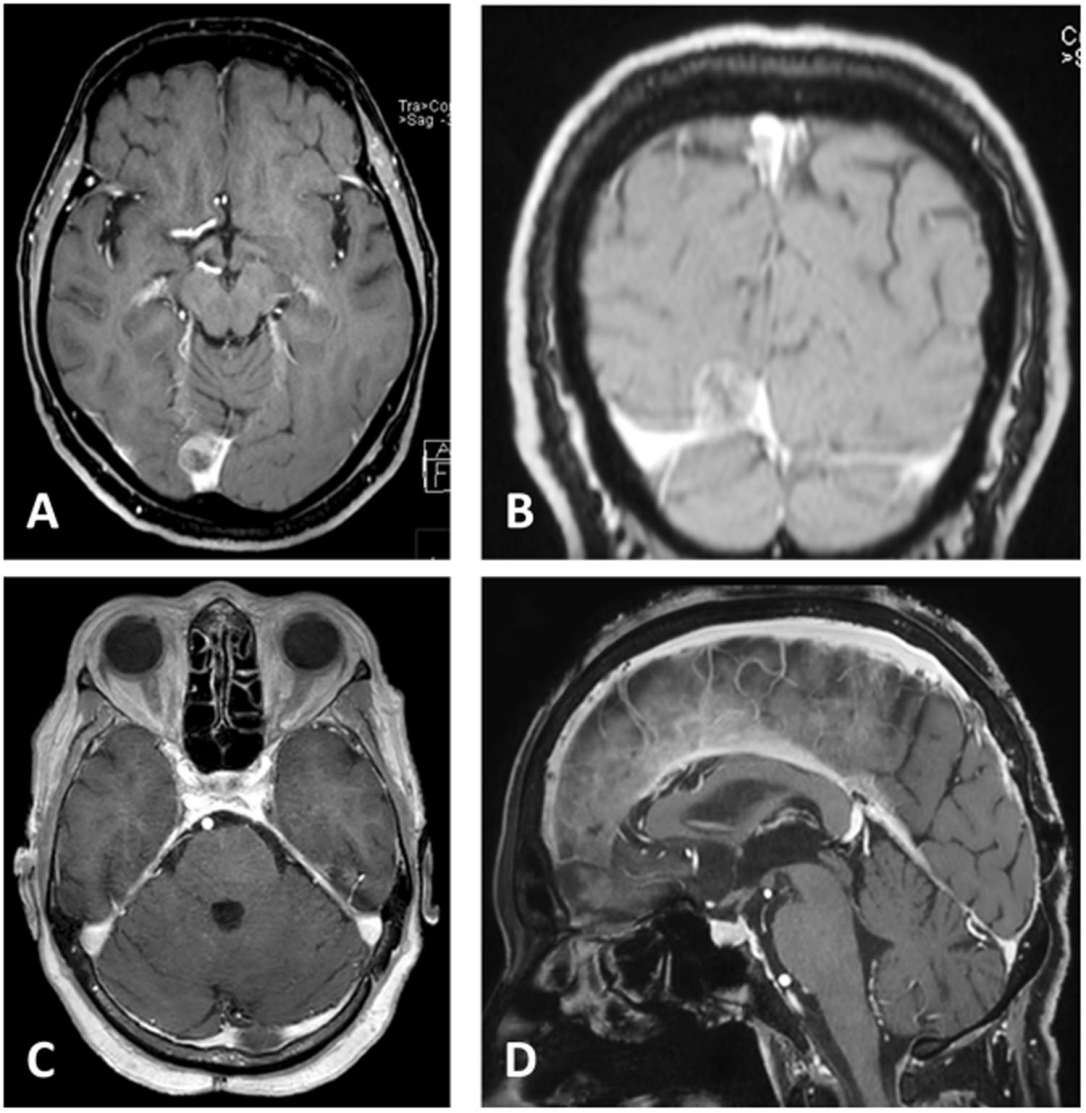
Incidental meningioma located in the right medial angle of the confluence in a 69-year-old female. Preoperative contrast-enhanced T1 MRI in axial (A) and coronal (B) reconstructions. The confluence was opened accidentally during resection of this rather small lesion that was intimately enclosed by the surrounding vessels. Imaging after resection (S2) via a midline parasagittal occipital craniotomy (C,D). The patient did not exhibit any deficit or complications postoperatively.
